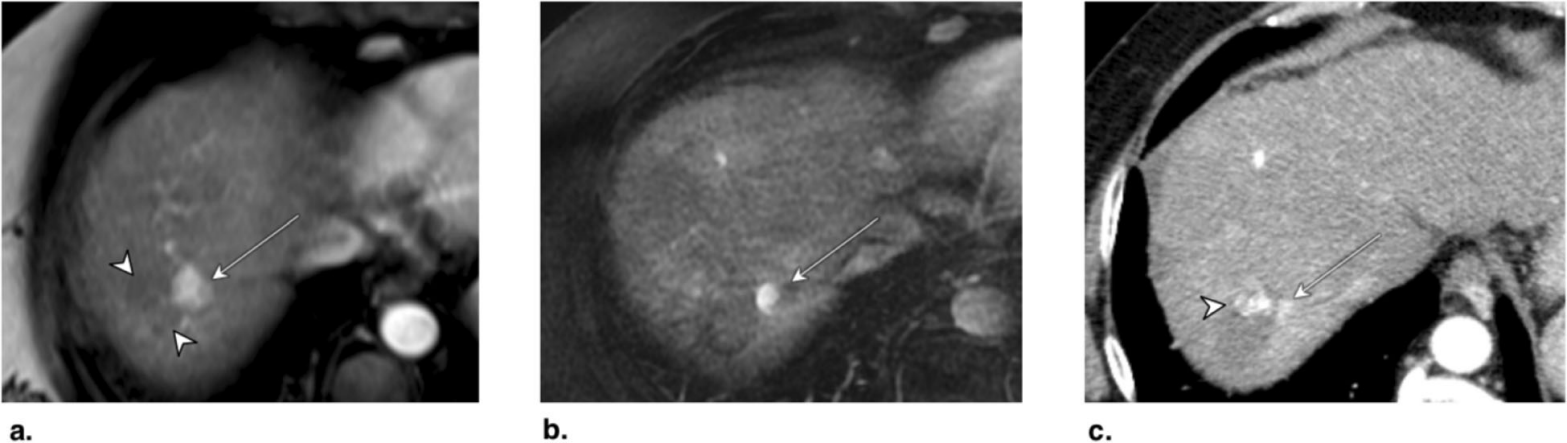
Axial T1-weighted MR in late arterial phase (AP) obtained (a) pretreatment: image shows recurrence (arrow) of a lesion previously treated with radiofrequency and transarterial chemoembolization (TACE) (arrowheads). b 2 months post stereotactic body radiotherapy (SBRT): image shows incomplete tumor regression. c Axial CT in late AP obtained 5 months post SBRT: image shows faint residual enhancement (arrow) that may represent expected enhancement pattern. The treated observation is categorized LR-TR equivocal. Note the ethiodized oil retention from previous TACE (arrowhead)
